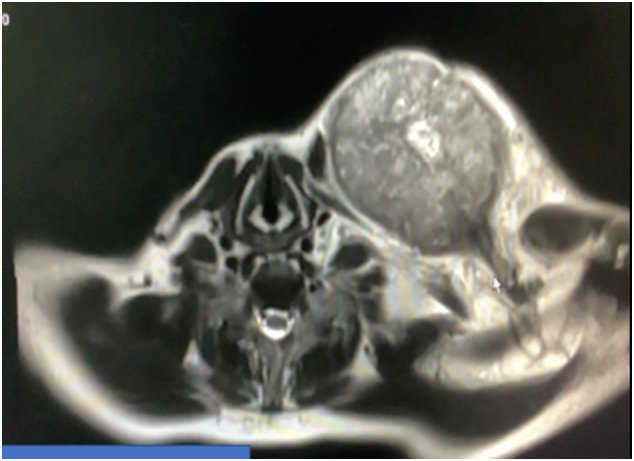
Contrast-enhanced MRI with huge clavicular mass.
